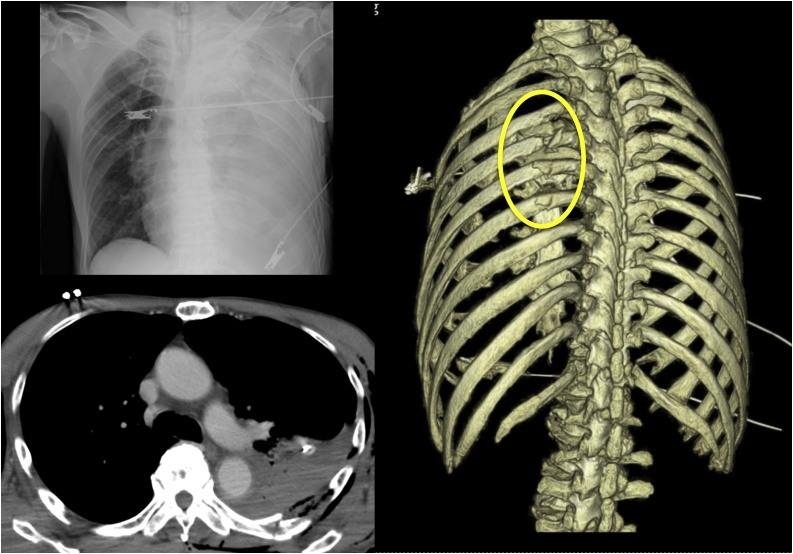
Chest radiography and computed tomography (CT) performed in a supine position on admission in the local hospital. Chest radiography and CT showed multiple left rib fractures and massive hemothorax. The fractured fourth to seventh ribs are shown on three-dimensional (3D) chest CT scan.
